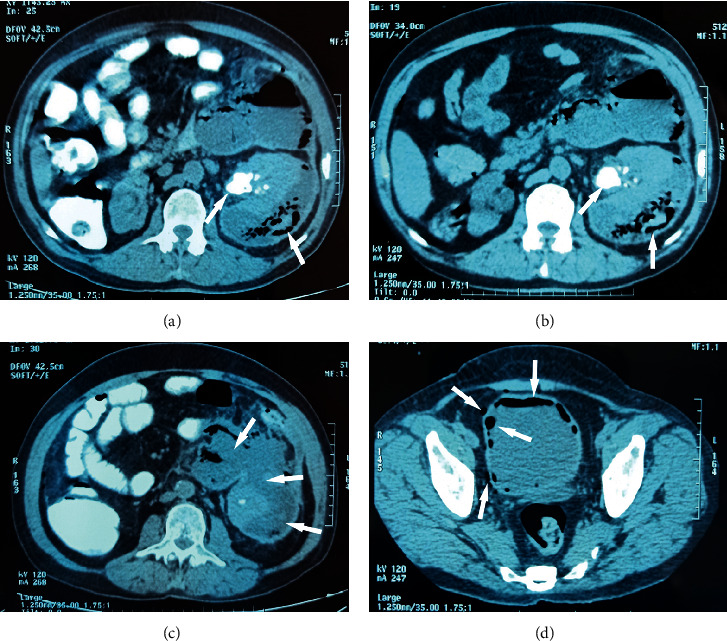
Abdominal CT scan showing (a, b) obstructive emphysematous pyelonephritis on a single functional kidney, (c) renal abscess communicating with intraperitoneal collections measuring approximately 208 × 113 mm with no passage of intraperitoneal contrast product at high digestive opacification, and (d) emphysematous cystitis.
